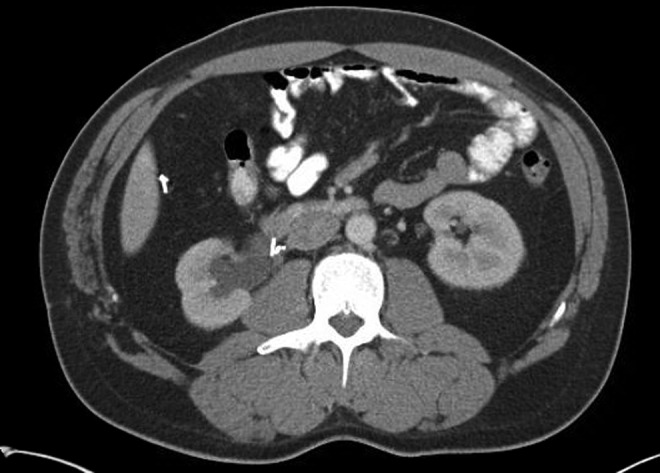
CT of the abdomen without contrast showing surgical clips near the ureteropelvic junction (UPJ). From this image, it is difficult to tell whether the clips are eroding into the ureter.
